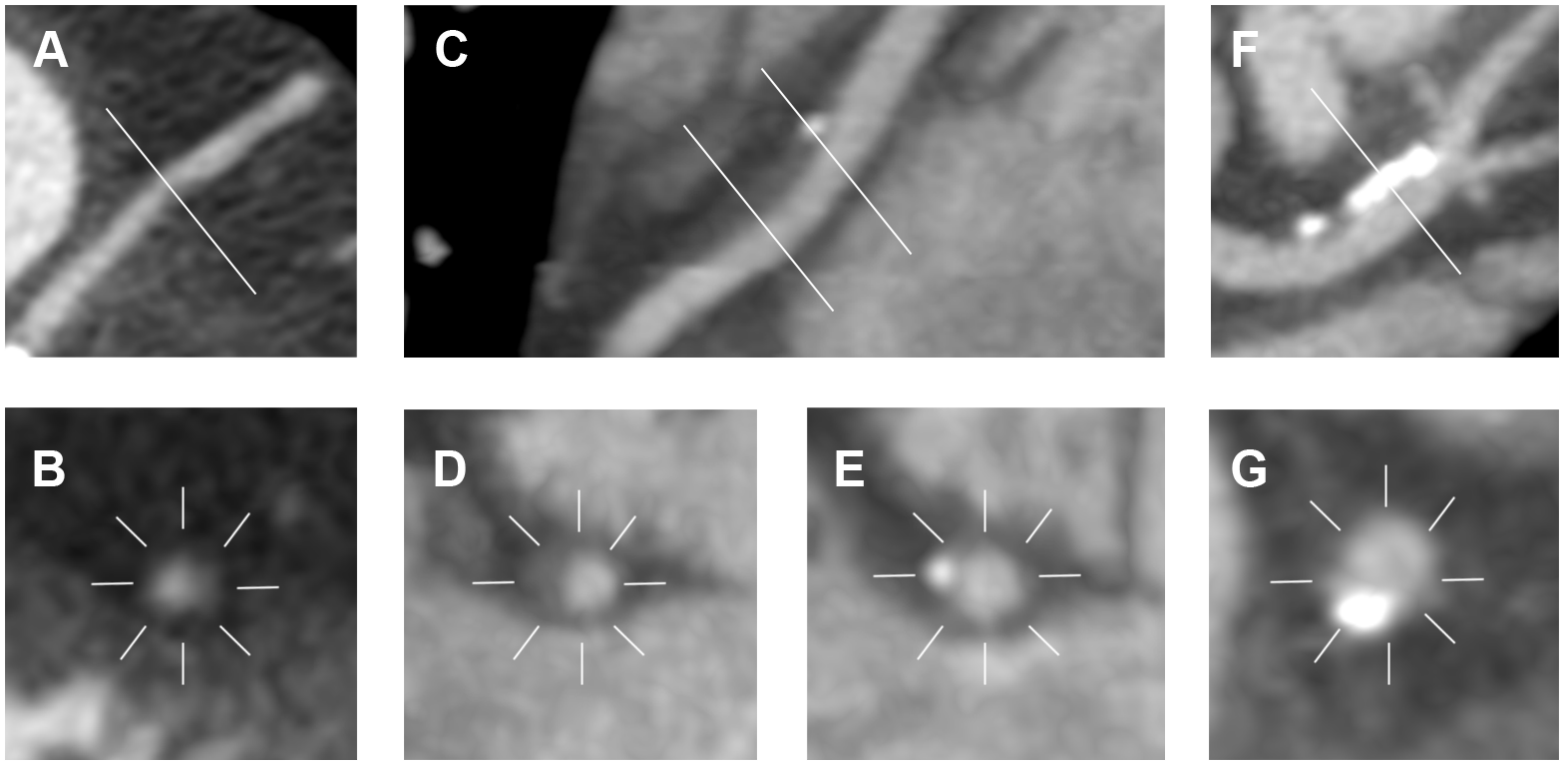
Different plaque types, as seen by cardiac computed tomography. A non-calcified plaque is shown in longitudinal and cross section (A and B). The degree of stenosis was 20–50%. A large mixed plaque is shown in longitudinal section (C) and in cross section at the level of non calcified (D) and calcified (E) components. A large calcified plaque is shown to the right. (F and G). The mixed and calcified plaques (C to G) were both eccentric in location and the degree of stenosis was <20%.
